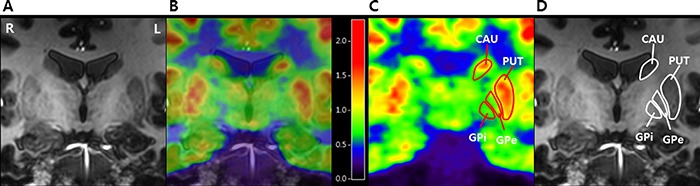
A 7-Tesla MRI image of the GPi and GPe (*A*), a^18^F-FDG PET/MRI fusion image (*B*), a^18^F-FDG HRRT-PET image with labeling (*C*), and selected regions of interest and labels that were based on the 7-Tesla MRI image (*D*). CAU: caudate nucleus; GPe: external globus pallidus; GPi: internal globus pallidus; L: left; PUT: putamen; R: right.
